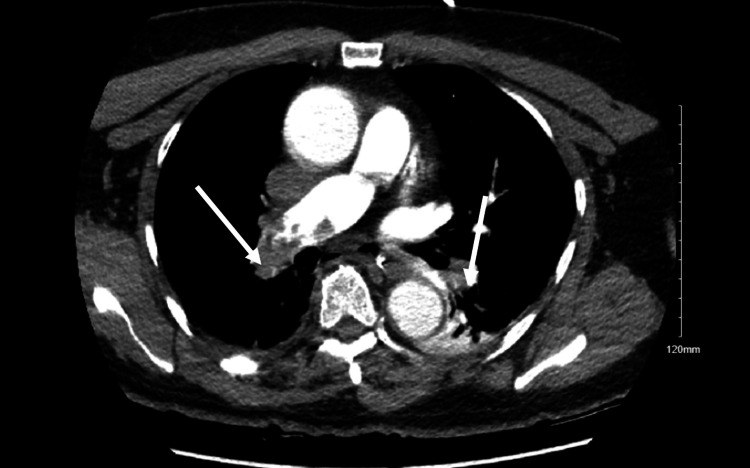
The CT pulmonary angiography shows bilateral filling defects within the pulmonary arterial tree, indicating extensive bilateral pulmonary emboli
